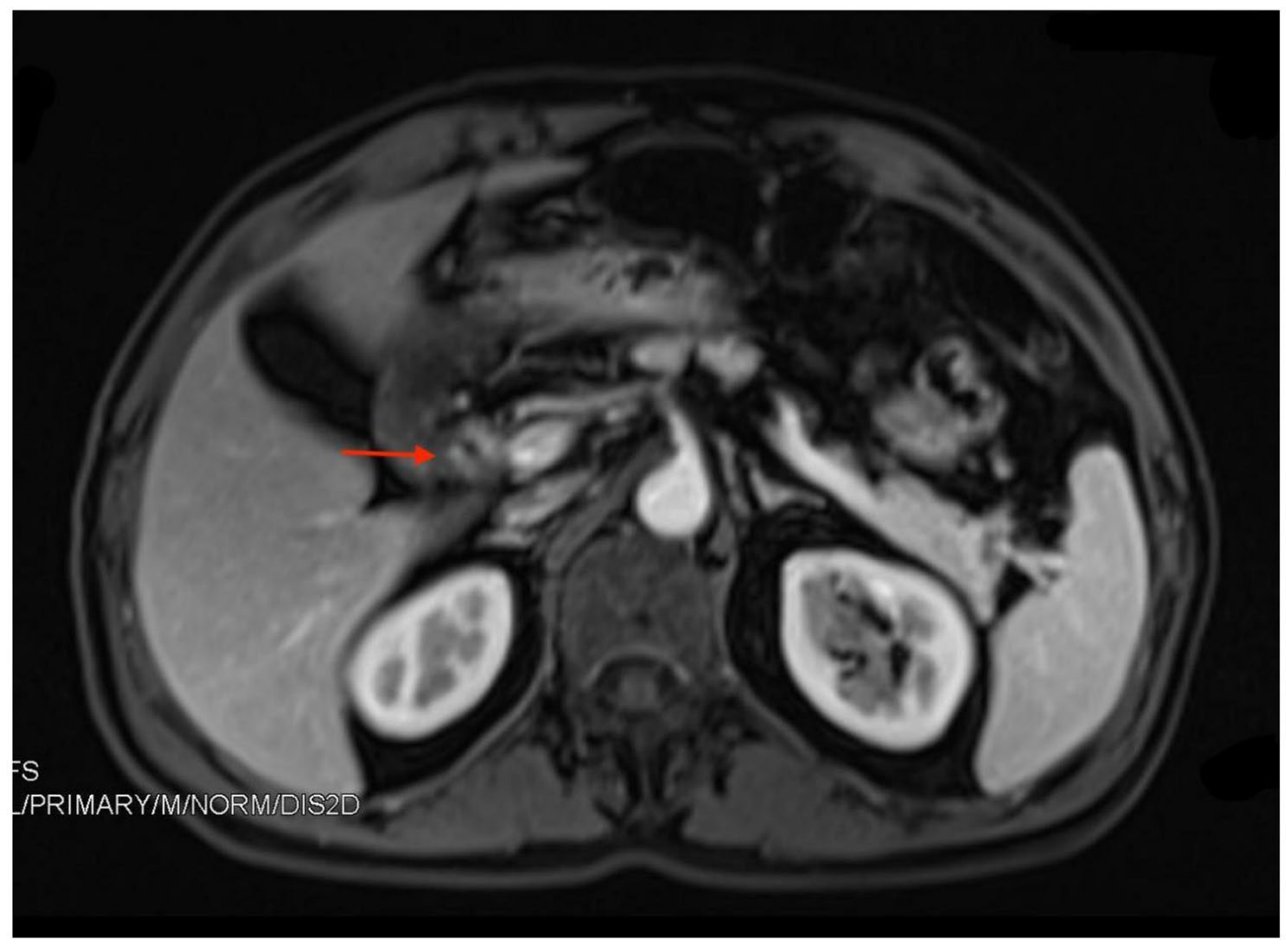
Heterogeneous thicken of the common bile ducts with contrast enhancement on T1-weighted images
